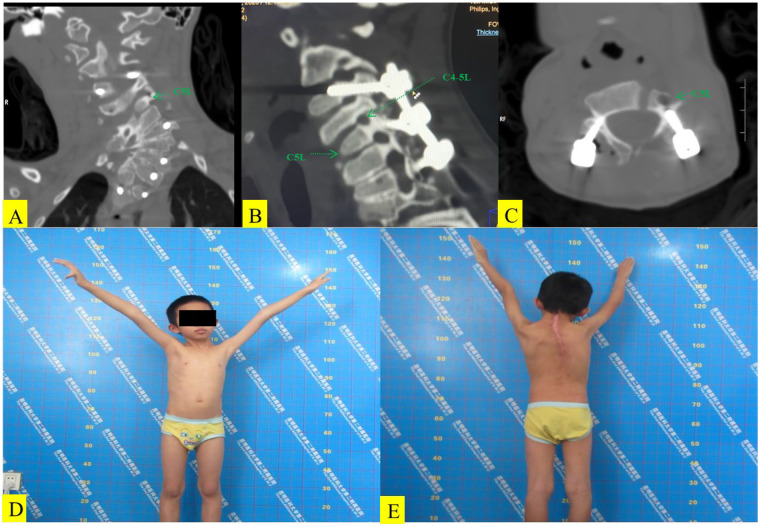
Postoperative CT image of the cervical spine reveals that the nail tract for internal fixation is satisfactory (A–C). At the 3-month follow-up, the myodynamia of the right deltoid muscle is normal (D,E).
